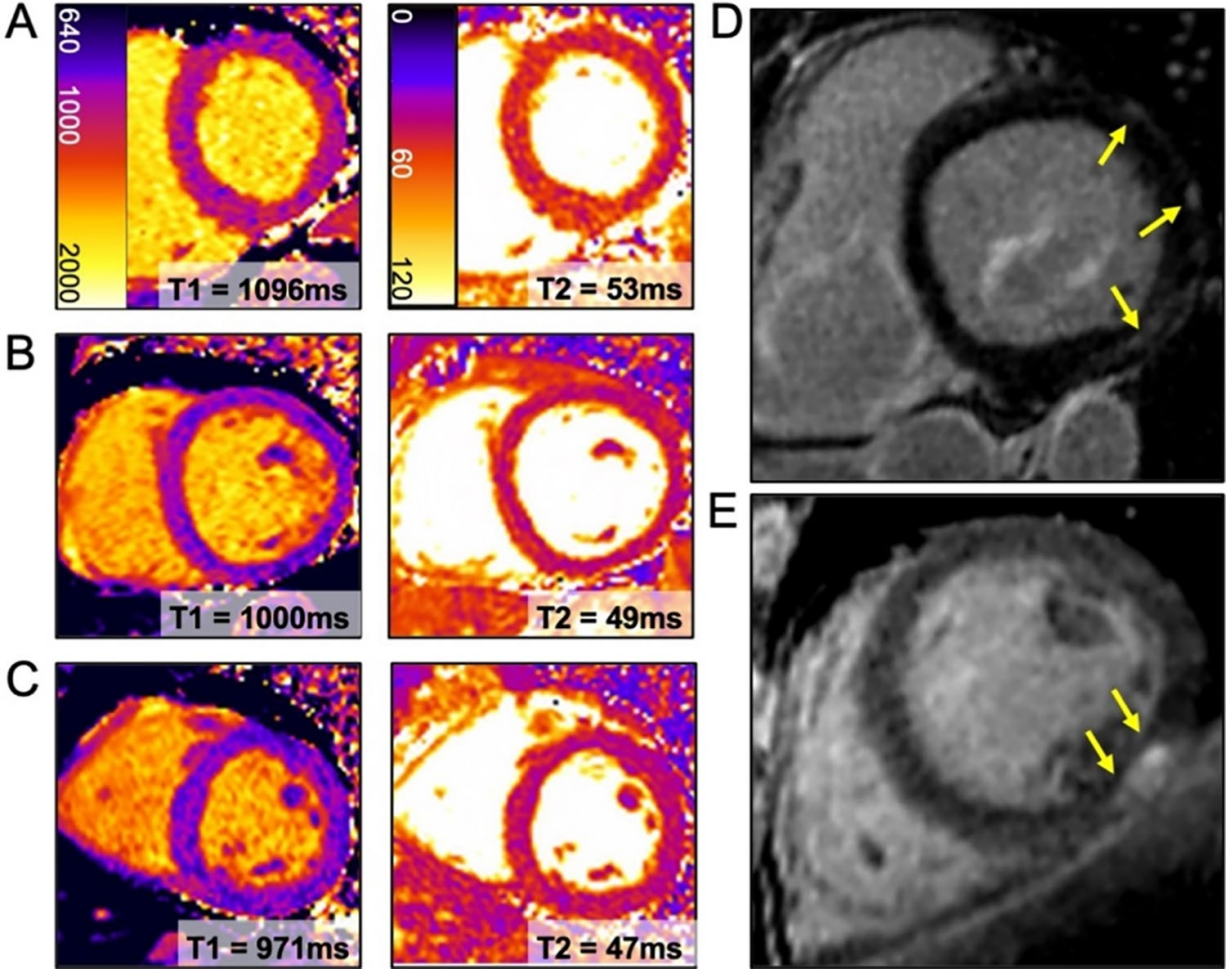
Representative Examples of cardiac MRI derived markers of diffuse fibrosis (T1 mapping) and edema (T2 mapping) (A-C) and focal fibrosis (late gadolinium enhancement) (D + E); A: Male patient with active ulcerative colitis and elevated markers of fibrosis and inflammation B: Male patient with Crohn’s disease in remission C: Male Healthy volunteer D-E: Non-ischemic scar (yellow arrows, areas of high signal intensity) in patients with Crohn’s disease (D) and Ulcerative colitis (E)
